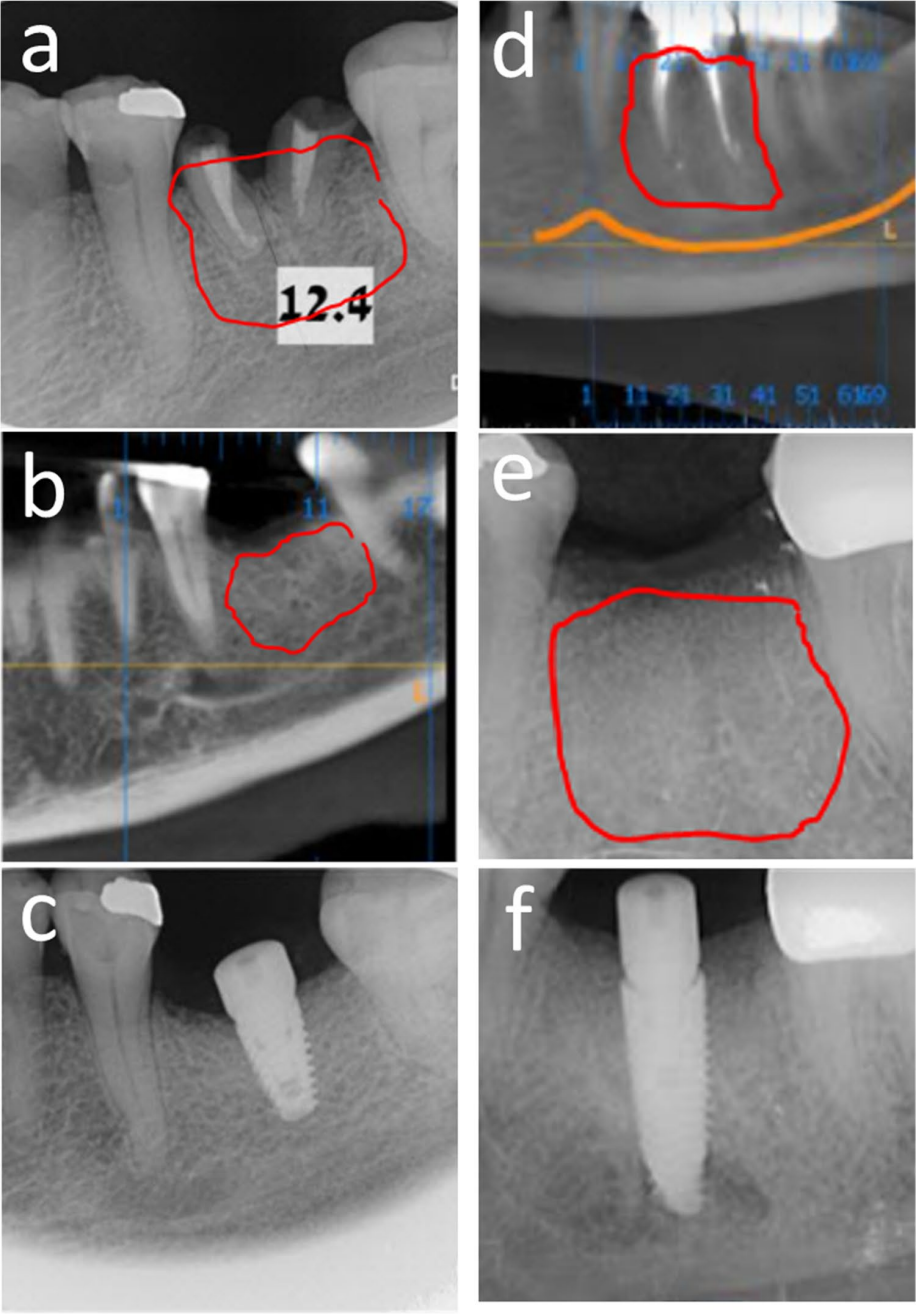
Example radiographs of graft sites. a Gen-Os® prior to extraction, b after grafting, and c after implant placement. d Ivory Dentin Graft™ prior to extraction, e after grafting, and f after implant placement
